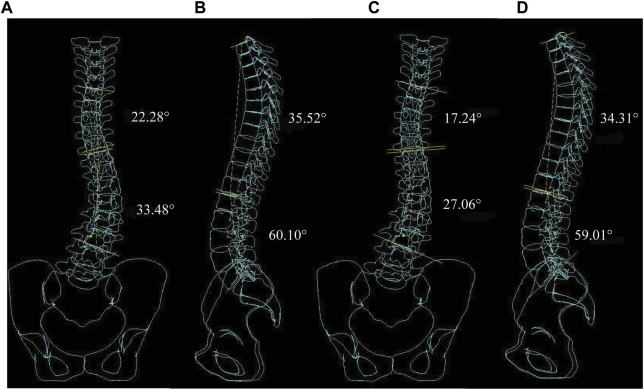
Virtual X-ray of normal spinal. (A) Coronal plane Cobb angle measurement in C1. (B) Sagittal plane Kyphosis and Lordosis angle measurement in C1. (C) Coronal plane Cobb angle measurement in C2. (D) Sagittal plane Kyphosis and Lordosis angle measurement in C2.
